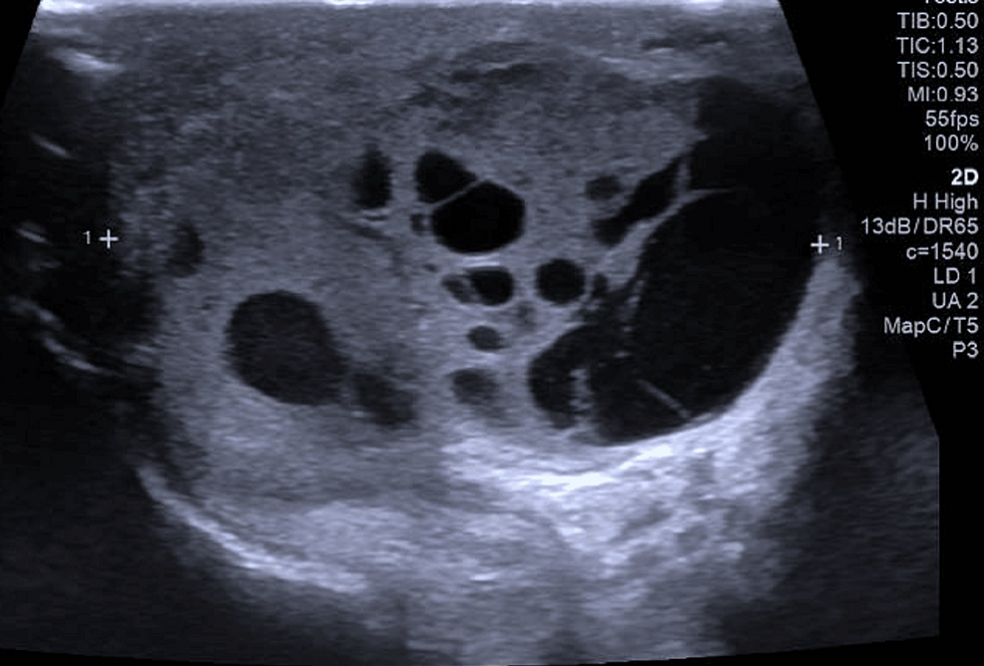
Ultrasound scan image showing a 44x27x37mm mixed solid/cystic mass with internal vascularity. within the right hemiscrotum.
